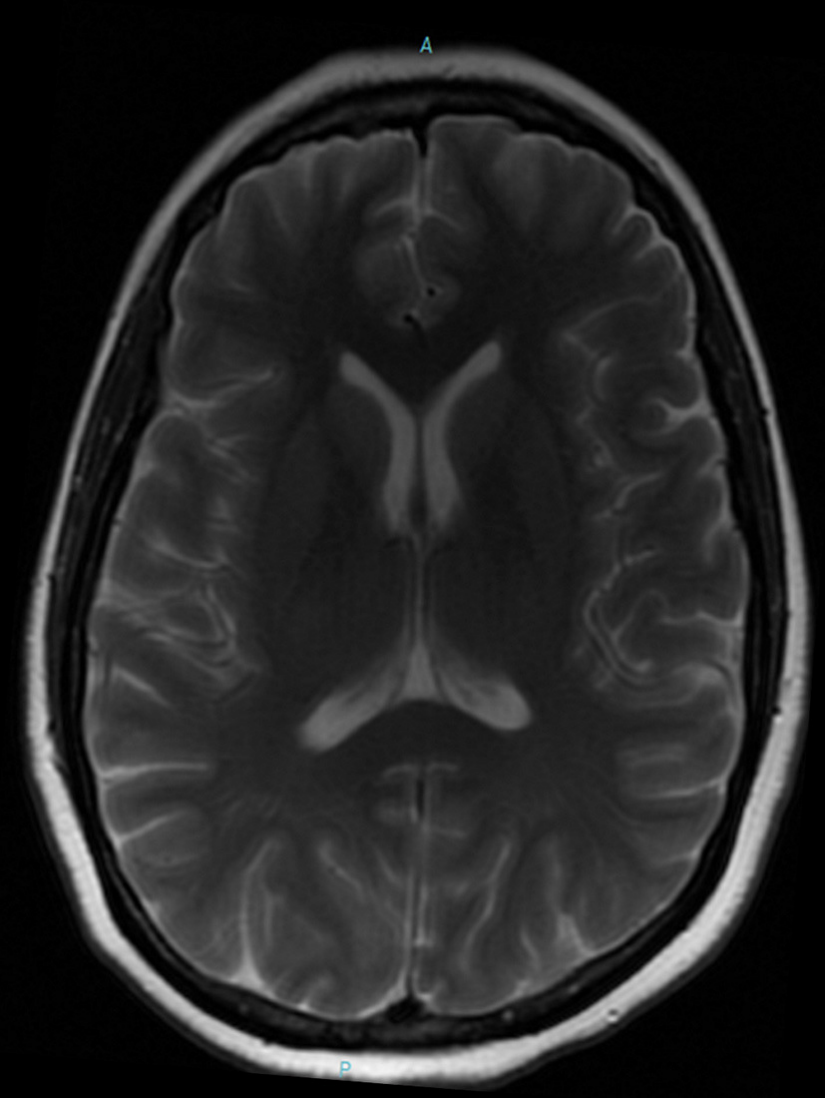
MRI T2 image of case 3 showing no brain abnormalities.
